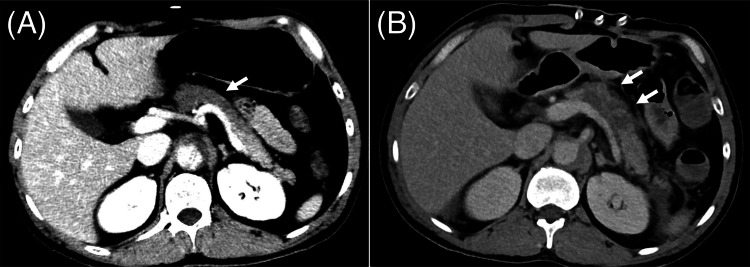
Retrospective review of pancreatic findings. Dynamic contrast-enhanced CT in the arterial phase (A) on the day of admission: When the window level is adjusted, a faint area of poor contrast is visible in the pancreatic body, which later develops into a pseudocyst. Contrast-enhanced CT in the equilibrium phase (B) on the third day: The poor contrast in the pancreatic body is more pronounced and is accompanied by increased density of the surrounding fatty tissue.
